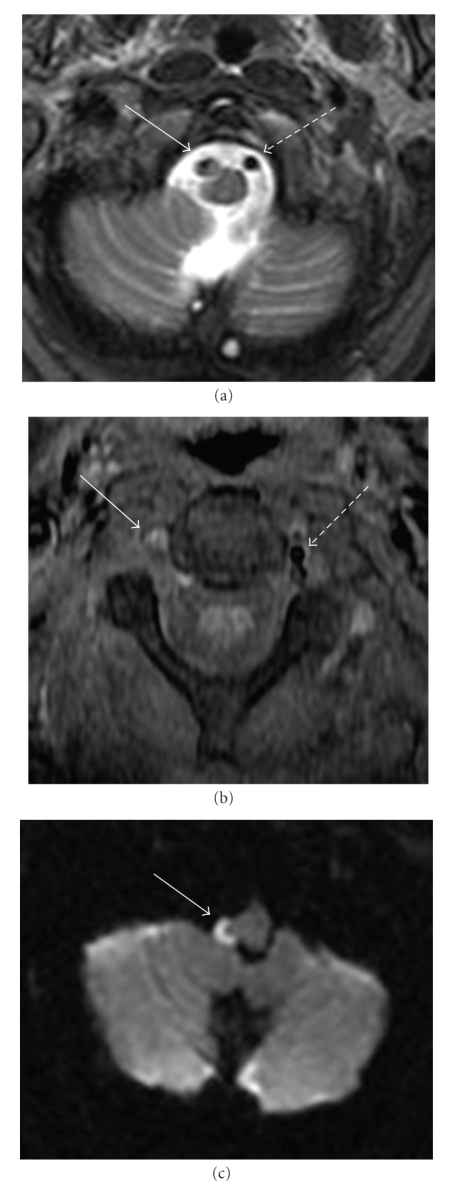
Brain MRI. (a) T2 sequence; a crescent-shaped filling defect is noted in the distal portion of the right VA (solid arrow). This lesion showed enhancement after intravenous infusion with gadolinium. (b) Fat-saturation sequence; a hyperintense lesion is noted in the third segment of the right VA (solid arrow). These findings are consistent with intramural clot formation and suggest vertebral dissection. For comparison purposes, the normal flow void in the left VA is shown (discontinued arrow). (c) Diffusion weighted image; a hyperintense lesion is noted in the right lateral medulla consistent with ischemic stroke.
